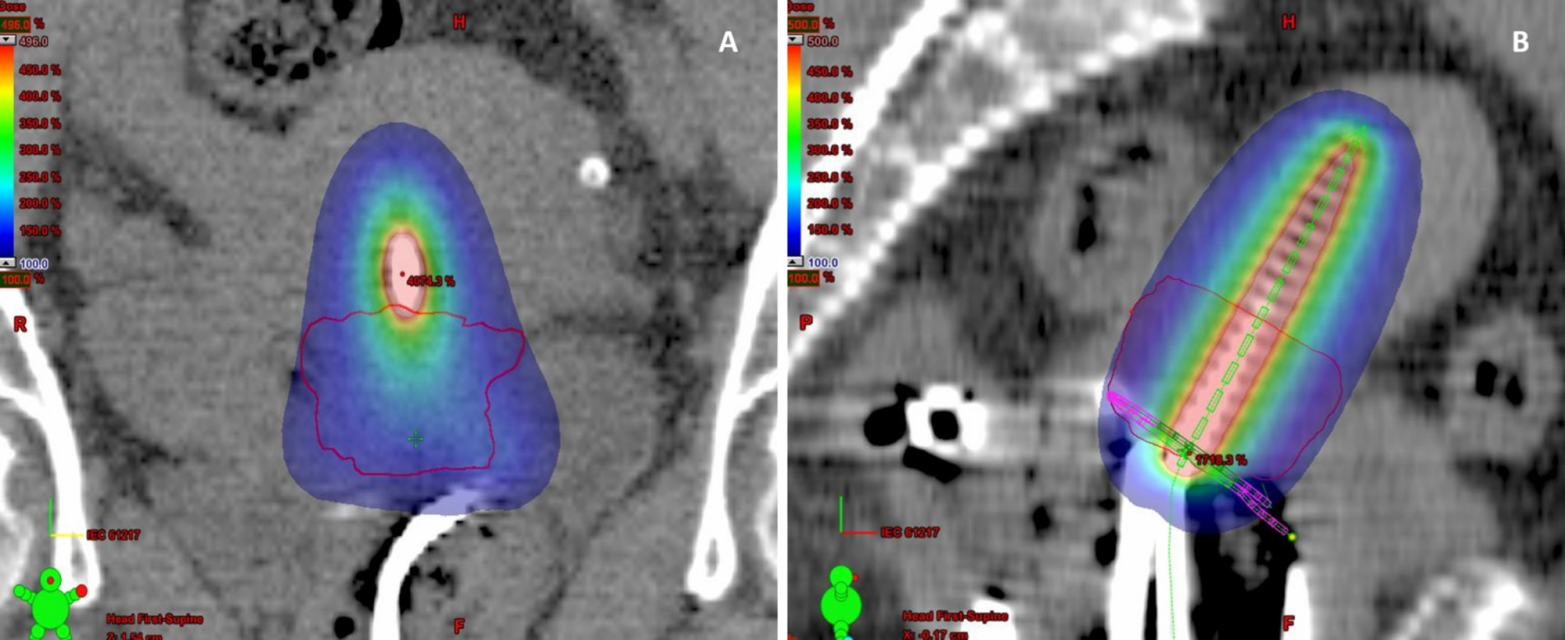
CT image (A, B) showing coverage of HRCTV (<25cc) by 100% isodose color wash HRCTV-High Risk Clinical Target Volume
